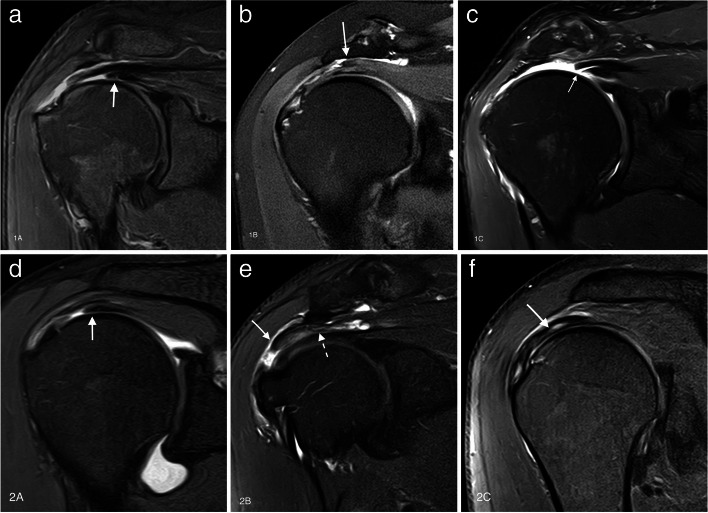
Delaminated tears of supraspinatus tendon. a–f Oblique coronal fat-suppressed PD-weighted MRI images. Full thickness delaminated tears. Articular layer more retracted than bursal layer (arrow in a). Bursal layer more retracted than articular layer (arrow in b) and bursal and articular layers equally retracted (arrow in c). Partial thickness delaminated tears. Articular side tear with intratendinous extension and retraction (arrow in d). Bursal side tear (arrow in e) with intratendinous extension (dashed arrow). Intrasubstance tear with horizontal intratendinous extension (arrow in f)
